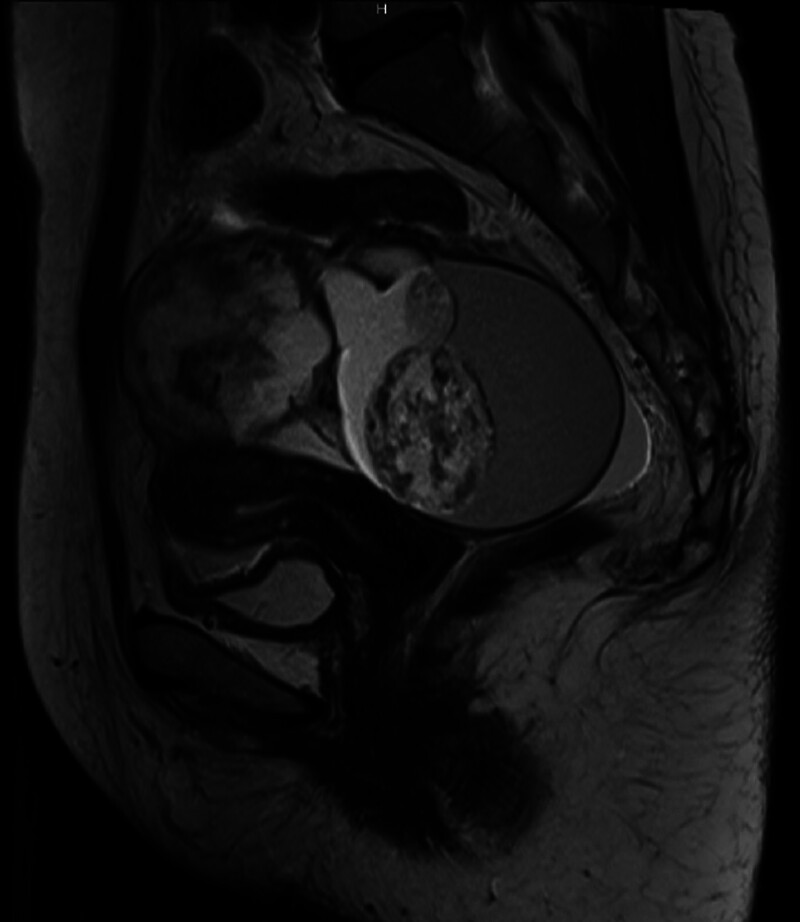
Sagittal T2-weighted MRI image. The enlarged cystic ovary contains solid components with high signal intensity.
